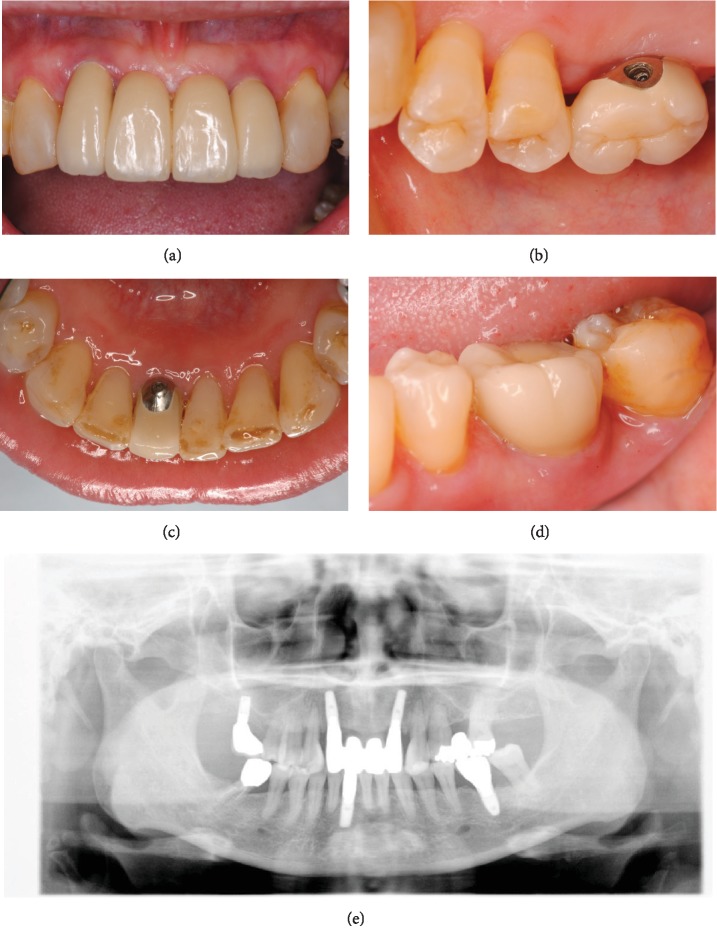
Clinical situation at the delivery of prosthetic rehabilitation: (a) at #12 and #22, (b) at #16, (c) at #41, (d) at #36, and (e) postoperative radiographs.
